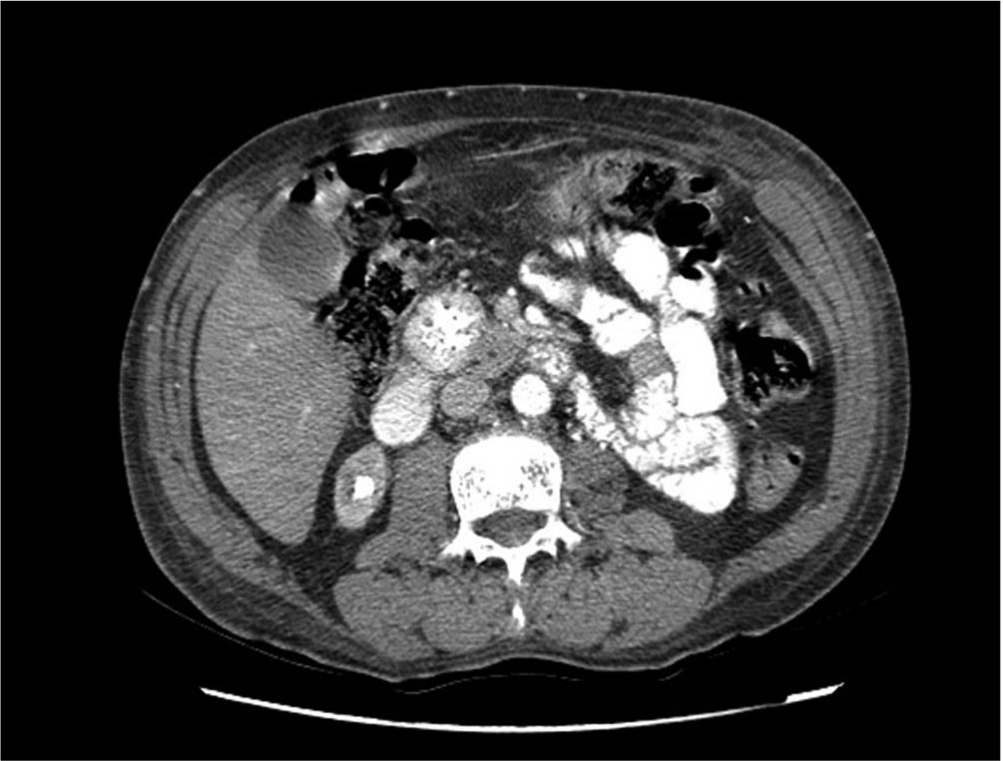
Triphasic computed tomography of the pancreas, arterial phase, showing a tumor in the head of the pancreas.
